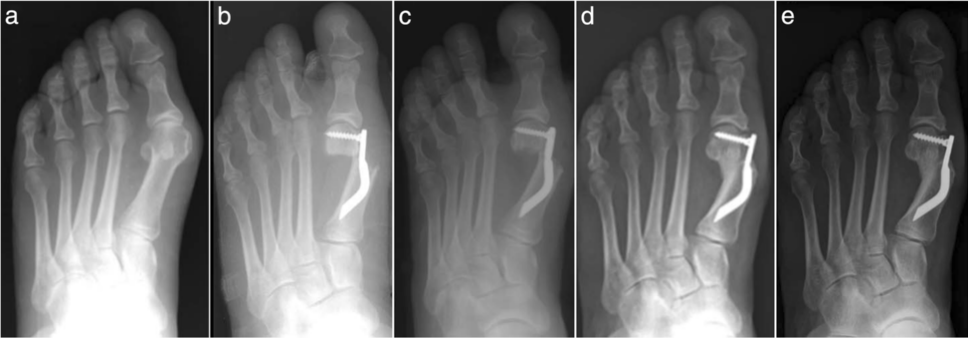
Case 5. A 52-year-old woman with moderate HV; antero-posterior radiographic images at: a Preoperative period. b 1-month follow-up. c 3-month follow-up. d 6-month follow-up. e 48-month follow-up showing the maintained correction of the deformity
